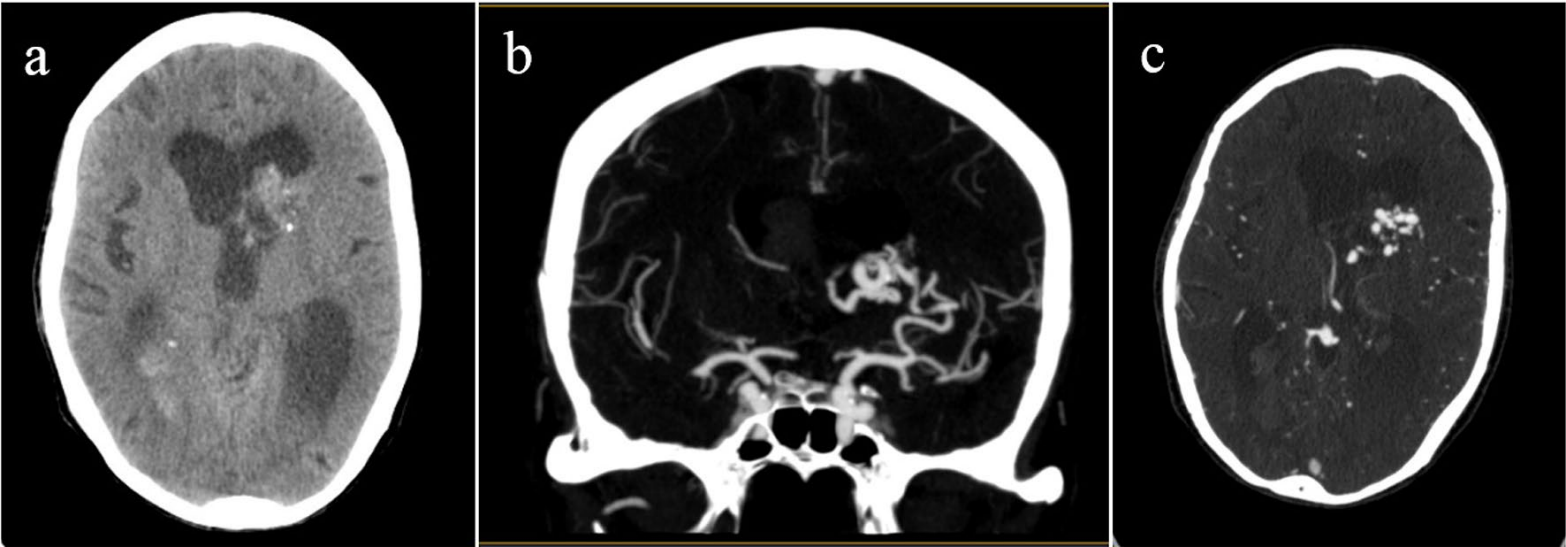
Non contrast CT scan (a) shows hyperdense lesion with small foci of calcification in the left caudate nucleus and intraventricular hemorrhage (IVH) in posterior horns of lateral ventricles. Coronal (b) and axial (c) cuts of brain CTA shows left caudate nucleus arteriovenous malformation (AVM) with feeding artery mostly from middle cerebral artery (MCA)insular branches and draining into deep veins.
